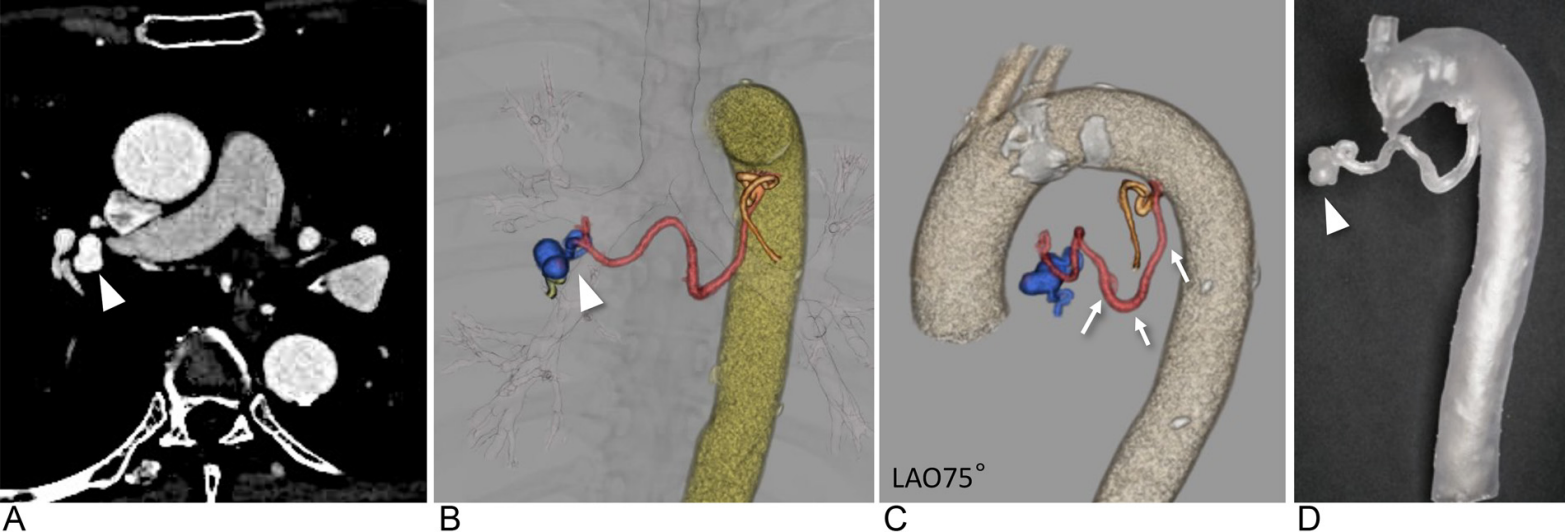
A 70-year-old female with a right bronchial artery aneurysm (Case 2). A right bronchial artery aneurysm (white arrowhead) is formed at the end of the right bronchial artery, which branches steeply from the inner curvature of the aortic arch and follows a tortuous and long course. (A) Computed tomography (CT) showing a 12 × 11 mm bronchial artery aneurysm (white arrowhead) located at the right hilum. (B and C) Volume rendering of CT angiography showing the right bronchial artery aneurysm (white arrowhead) and a white arrow showing the right bronchial artery. (D) Transparent and flexible patient-specific hollow vascular models are used for the right bronchial artery aneurysm (white arrowhead).
